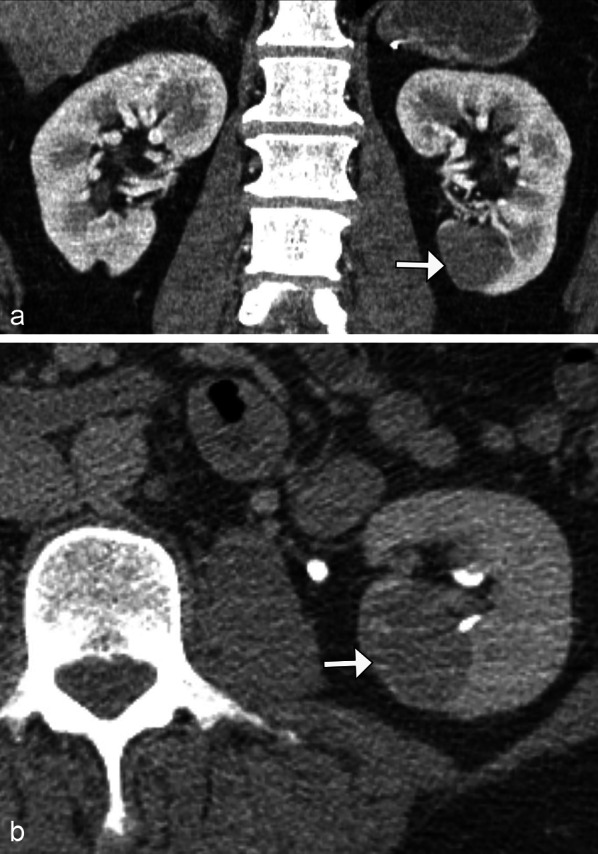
Papillary renal cell carcinoma.* Coronal (a) and axial (b) contrast CT images demonstrate a hypoenhancing left lower pole renal mass (arrows), which was biopsy confirmed as papillary renal cell carcinoma mimicking the solitary hypoenhancing mass of lymphoma
